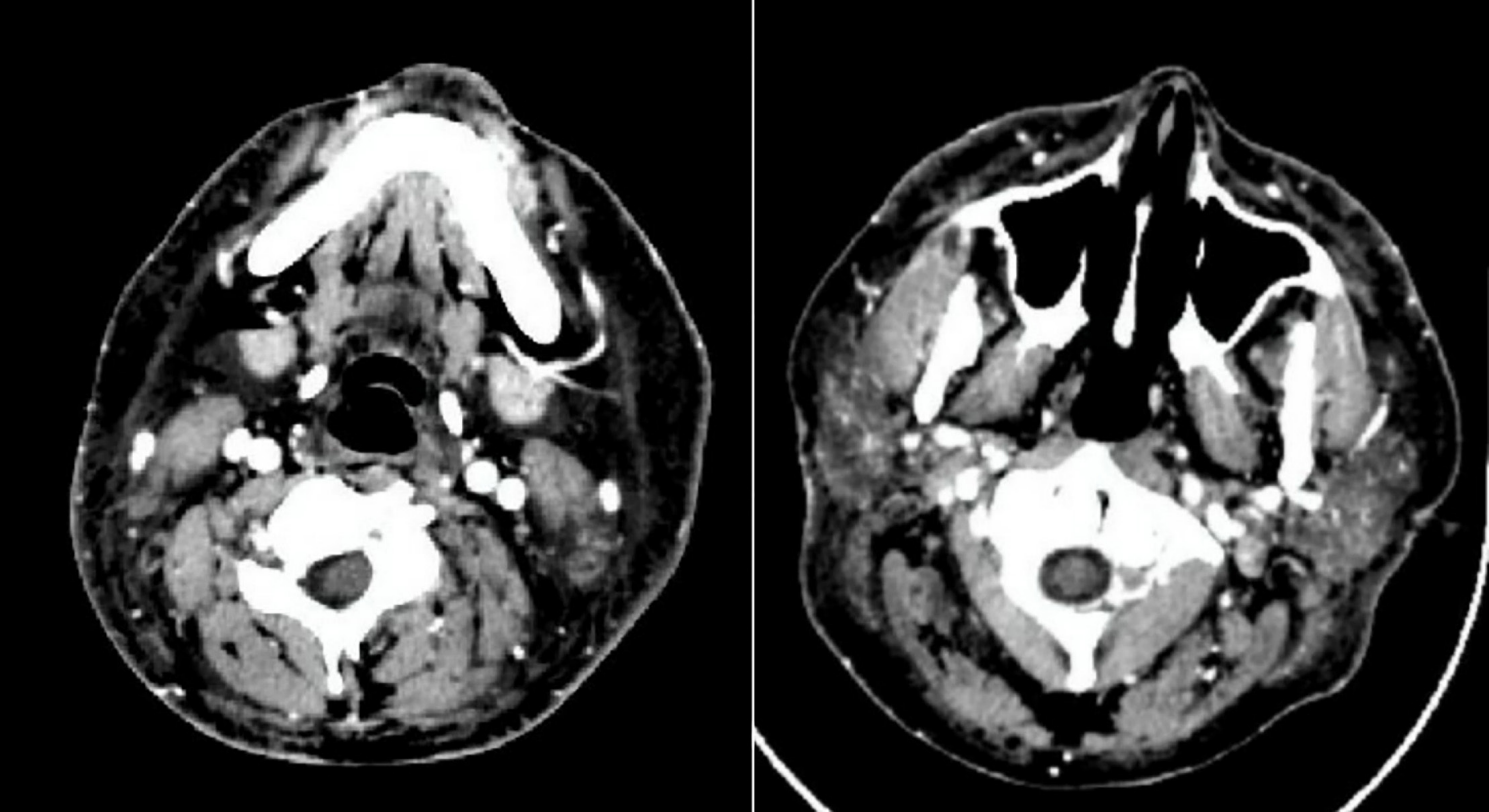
A contrast head and neck CT scan (axial view) confirming no mass or thrombus, yet subcutis and muscle swelling in the head and neck regions were evident.
